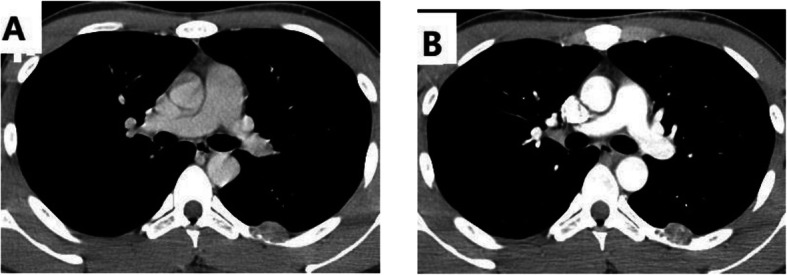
Chest CT showed that there was a tumor located the 6th posterior left rib and bony cortex erosion (A). Enhanced CT could saw the tumor non-uniformity enhancement (B)
